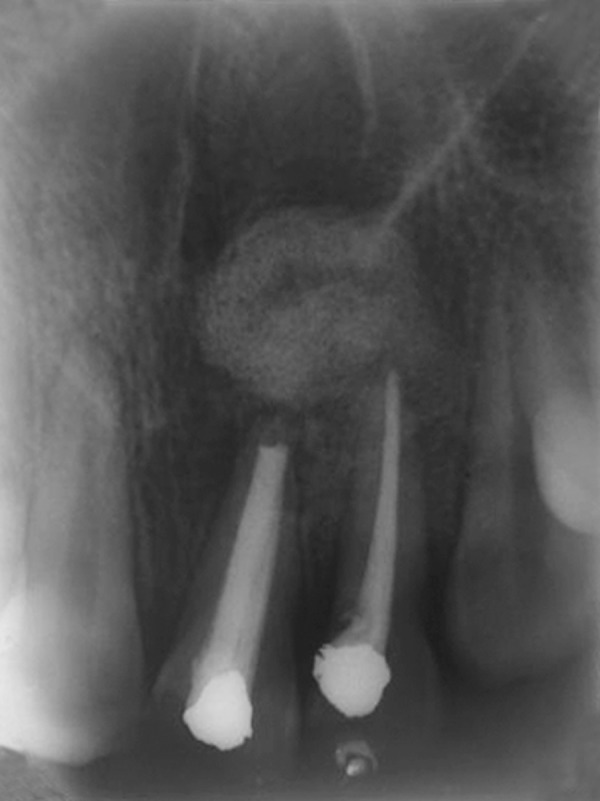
Three months postoperative X-ray
